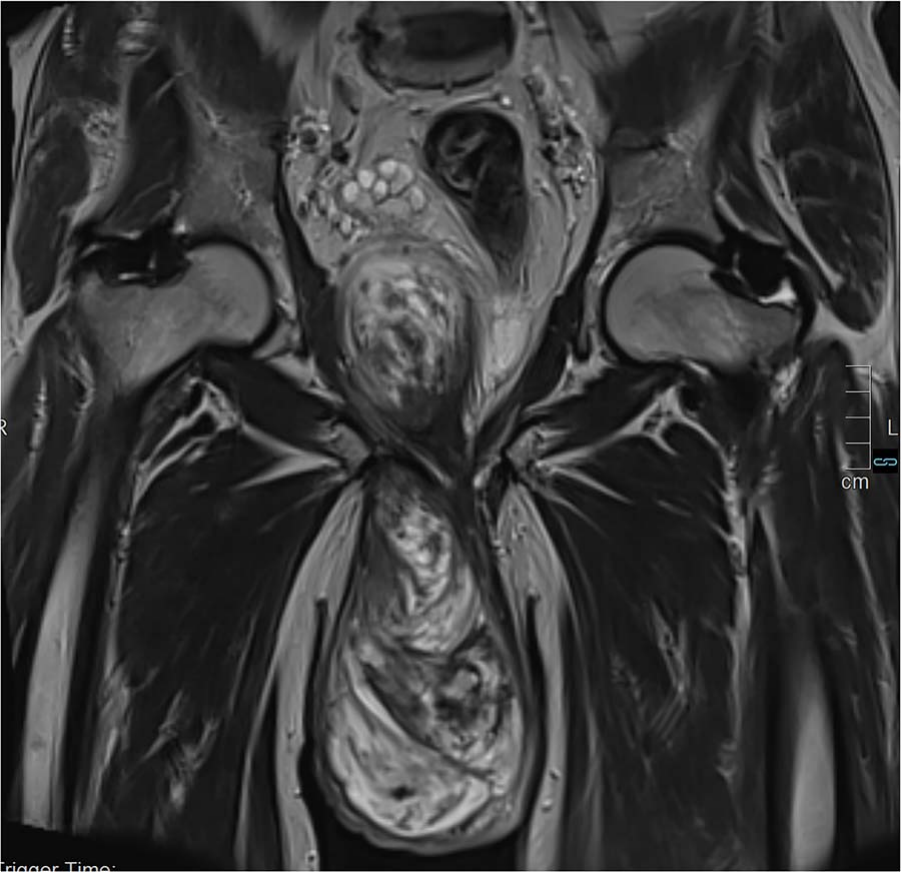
Coronal MRI section demonstrating the extent of infiltration and endopelvic extension.
